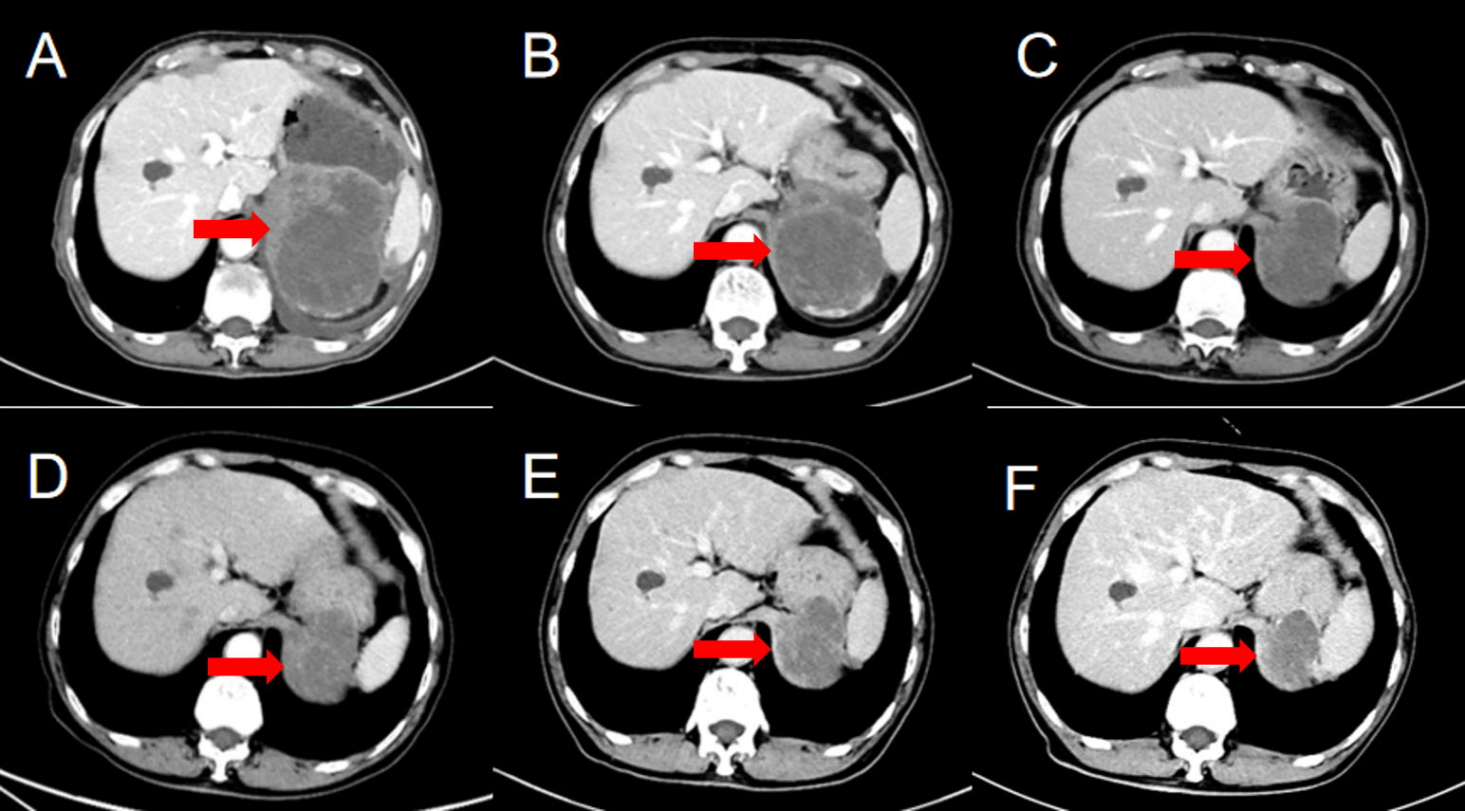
Computed tomography images before and after treatment. Review after immunotherapy showed significant reduction in the sizes of the left adrenal region. The time of CT was (A) 2021-07-30, (B) 2021-09-13, (C) 2021-11-30, (D) 2022-02-20, (E) 2022-05-12, (F) 2022-08-09.
